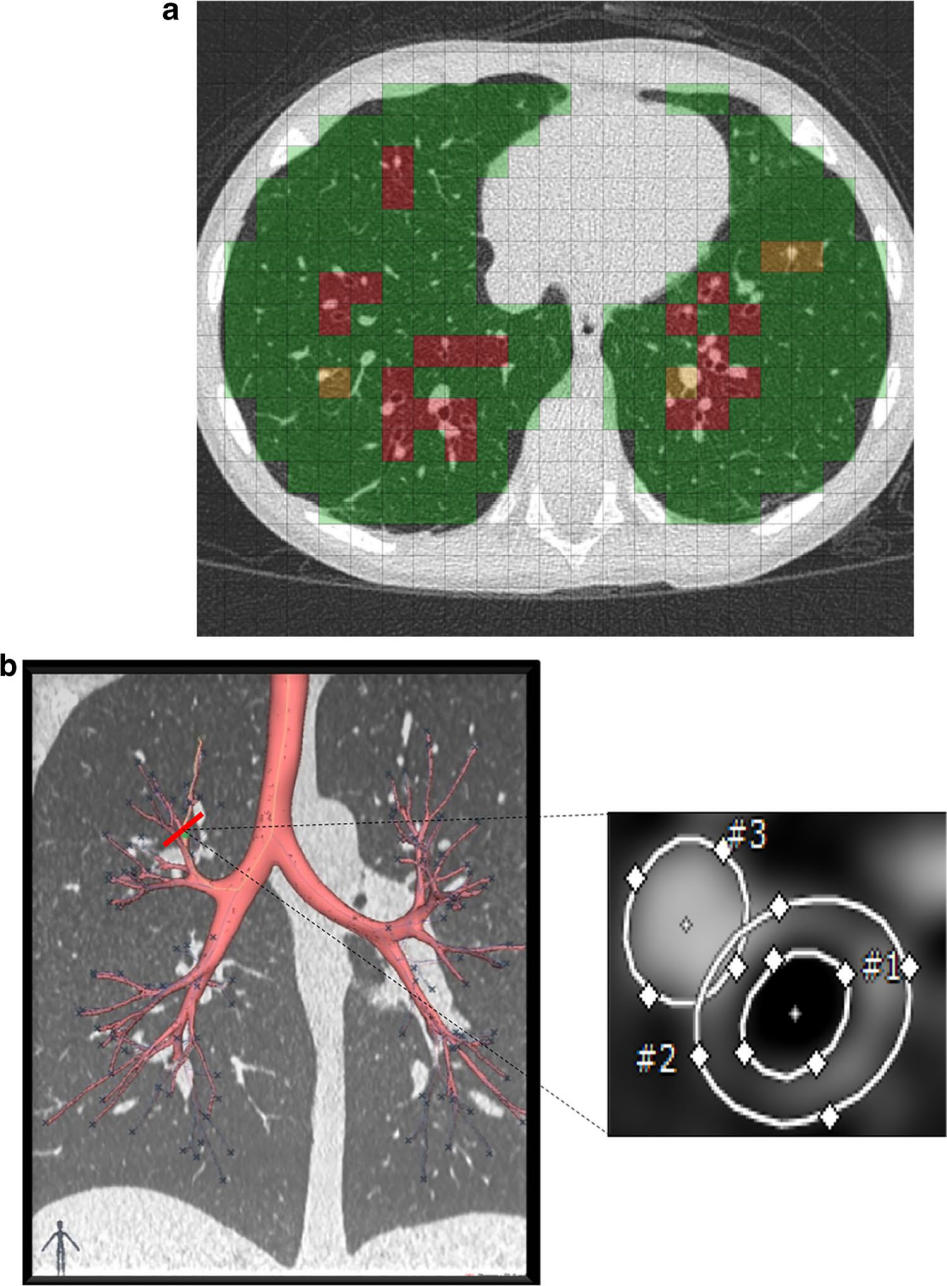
Advanced image analysis of CT in a 12-year-old boy with cystic fibrosis (CF). a, b PRAGMA (Perth-Rotterdam Annotated Grid Morphometric Analysis) CF score (a) and airway–artery (AA) ratio (b). The PRAGMA-CF score is a scoring system that uses a grid to quantify all typical CF lung disease components. In the example, green colour is used for normal lung tissue, red for bronchiectasis and yellow for bronchial wall thickening. The percentage of disease (%Dis) is the total score of these components expressed as a percentage (%) of the total lung volume. In the AA ratio, the entire bronchial tree is automatically segmented (b) along with the adjacent pulmonary arteries. Each AA pair is then segmented (perpendicular view of airway and artery measurements) to compute several parameters, such as percentage of bronchiectasis, bronchial wall thickening and lack of tapering. Both PRAGMA-CF and the AA method have been automated using artificial intelligence techniques and are validated
